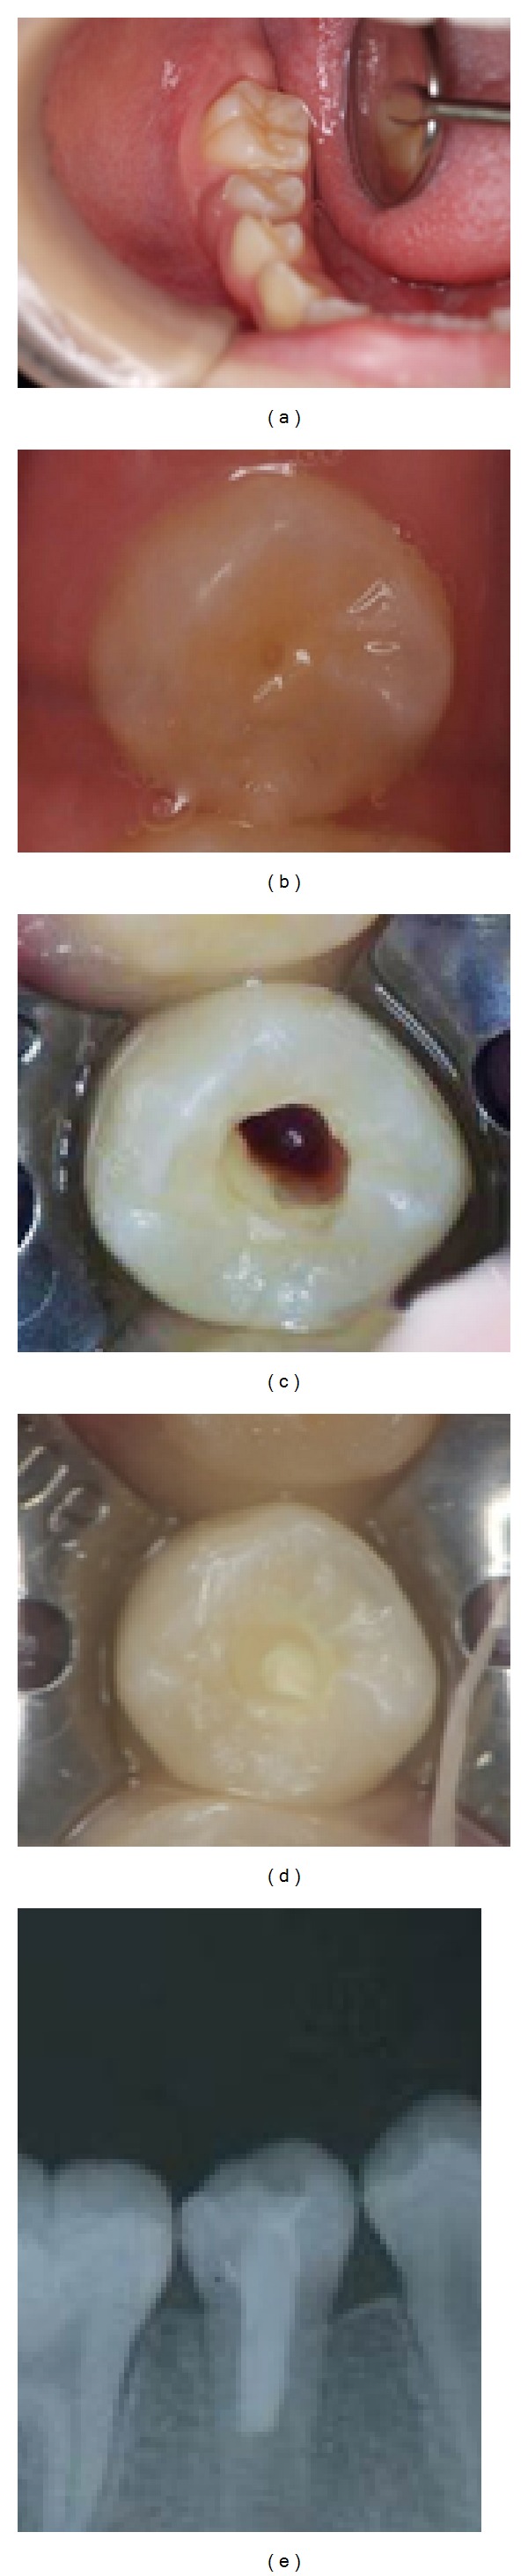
(a) Intraoral photograph showing no abnormalities of gingiva. (b) The central cusp of the mandibular right second premolar had been fractured. (c) After controlling hemorrhage, viable tissue was observed in the canal because insertion of a K-file evoked a sensation. (d) Placement of MTA in the canal. (e) Postoperative X-ray photograph showing MTA placement in canal.
